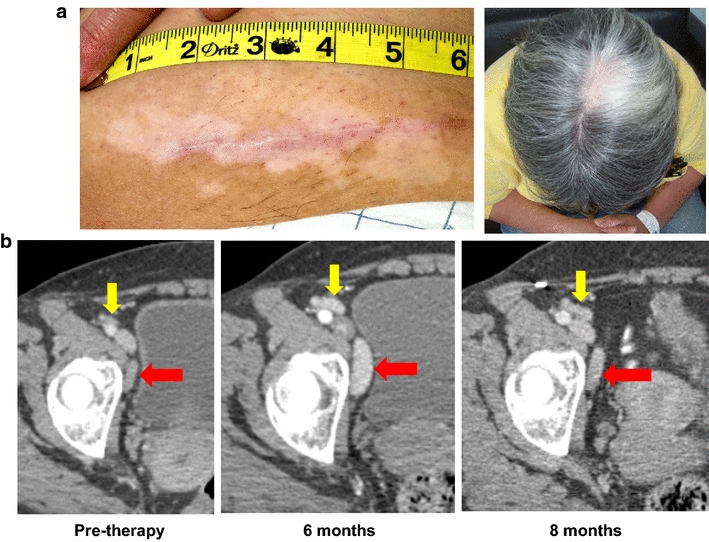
Transient melanoma progression in a patient treated in Cohort A, accompanied by vitiligo. a This patient developed the onset of vitiligo and greying hair after receiving four cycles of Melanoma GVAX. Vitiligo was initially confined to a prior lymphadenectomy incision site, but progressed to other skin sites and hair over the next few months. b Pelvic CT scan at the 6-month evaluation interval showed enlarging left pelvic lymph nodes (*yellow* and *red arrows*), biopsy-proven to be recurrent melanoma. At 8 months, these lesions had regressed without further intervention. Two months later, they enlarged again and the patient went on to receive other therapies.
